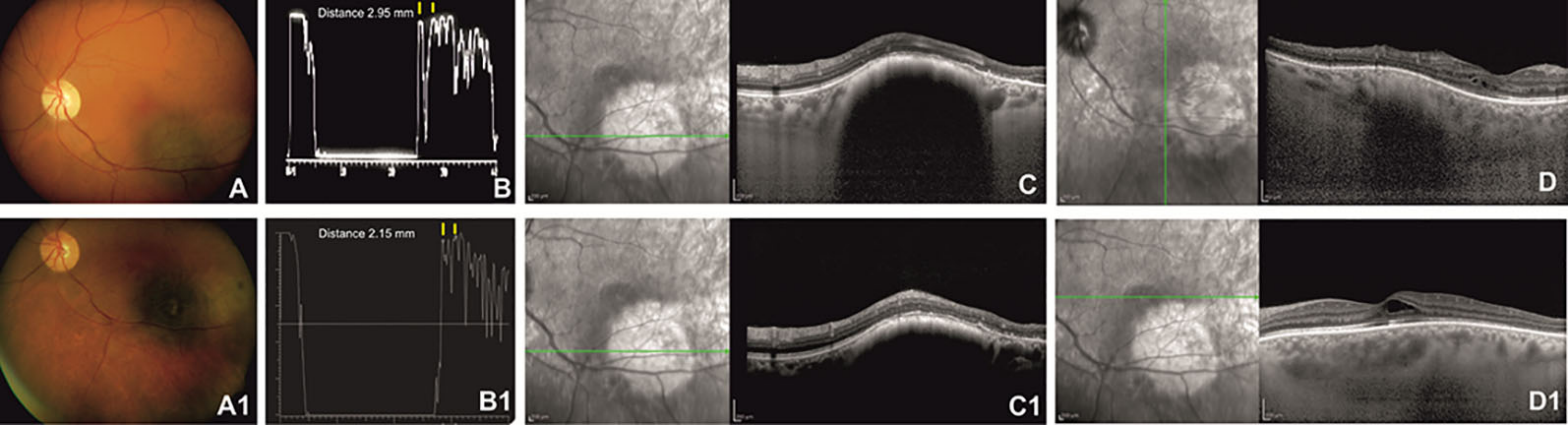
Left eye of a 62-year-old patient affected by choroidal melanoma before ruthenium-106 brachytherapy (top row). Color fundus image shows an elevated and yellow lesion located at the posterior pole (A). A-scan echography shows low reflectivity. The yellow arrows over the peaks of the two high and perpendicular spikes, as shown in the echogram, indicate a tumor thickness of 2.95 mm (B). Spectral domain–optical coherence tomography (SD-OCT) B-scan over the lesion reveals a highly reflective band within the choriocapillaris layer with posterior shadowing (C) and normal central retinal thickness with rare intraretinal cysts in the macular region (D). Color fundus image shows the same tumor after ruthenium-106 brachytherapy (A1). At A-scan ultrasound, the lesion presented high reflectivity with a tumor thickness of 2.15 mm (B1), confirmed also by the SD-OCT B-scan (C1) that shows in the macular region an increased central foveal thickness with intraretinal cysts due to the radiation maculopathy (D1).
